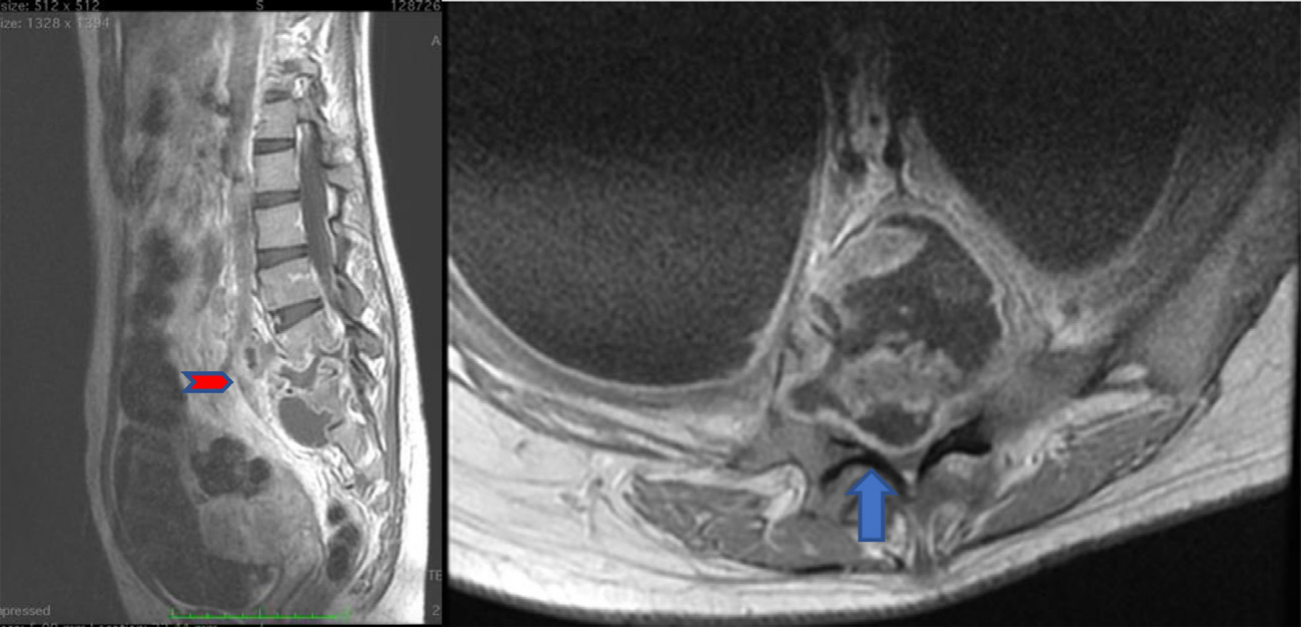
Contrast-enhanced MRI: (A) sagittal plane showed destruction of the discus and superior endplate corpus vertebra of L4 and L5 with an intraosseous abscess in the L4 and L5 corpus vertebral, expanding to the anterior subligamentous (red arrow); prevertebral abscess, right and left paravertebral abscess, and retro vertebral abscess; (B) the axial plane showed the retro vertebral abscess and granulomatous tissue in the thecal sac and compacted cauda equina resulting in severe central canal stenosis (blue arrow)
